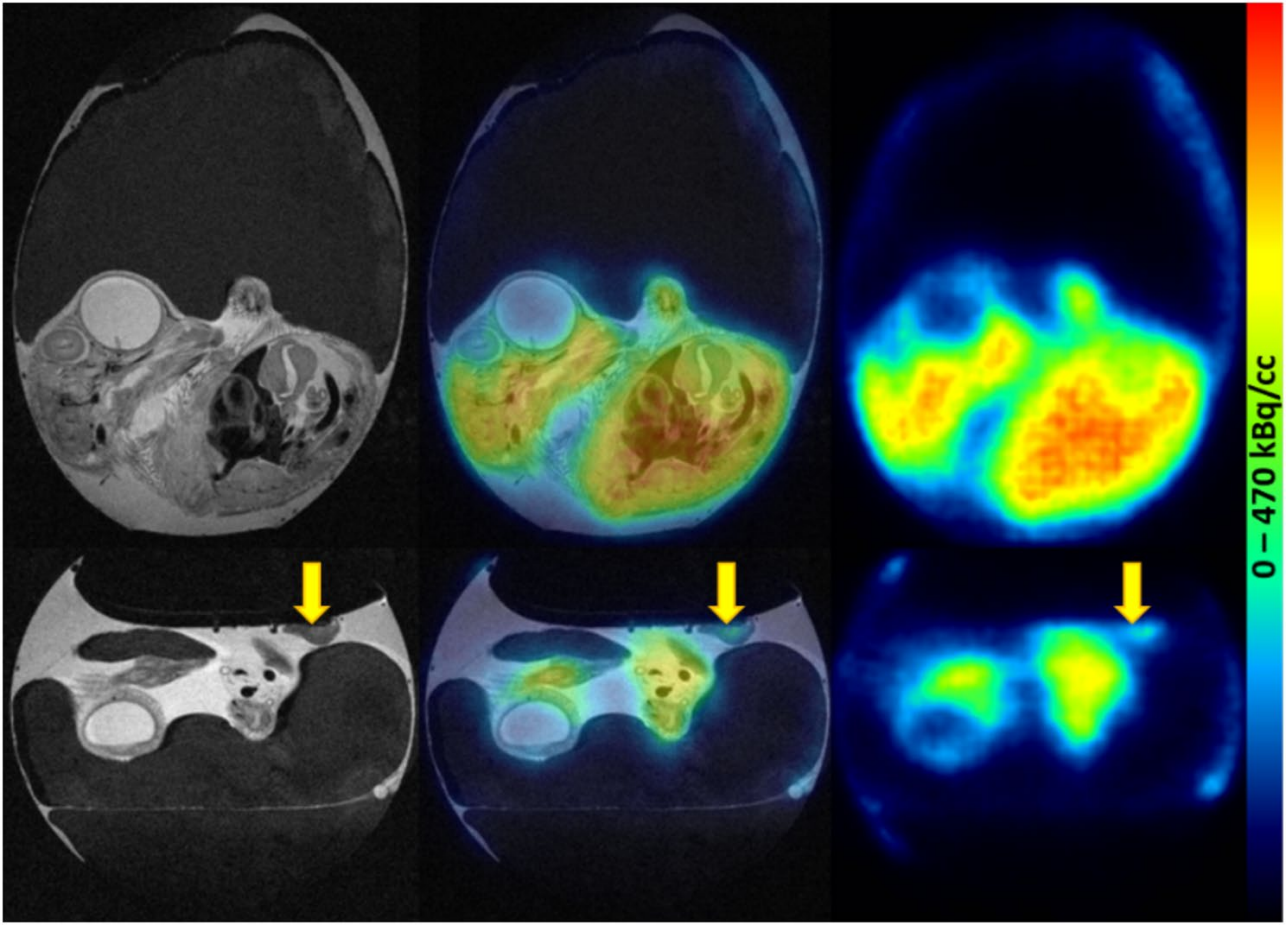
Representative images of [^68^Ga]Ga-Pentixafor distribution in the fertilized chicken egg on EDD16. PET scan was started 60 min p.i. of 7.19 MBq [^68^Ga]Ga-Pentixafor. Left: T2-weighted TurboRARE images, right: PET images, middle: fused PET/MR images. The xenograft (HCT116) is marked with yellow arrows
